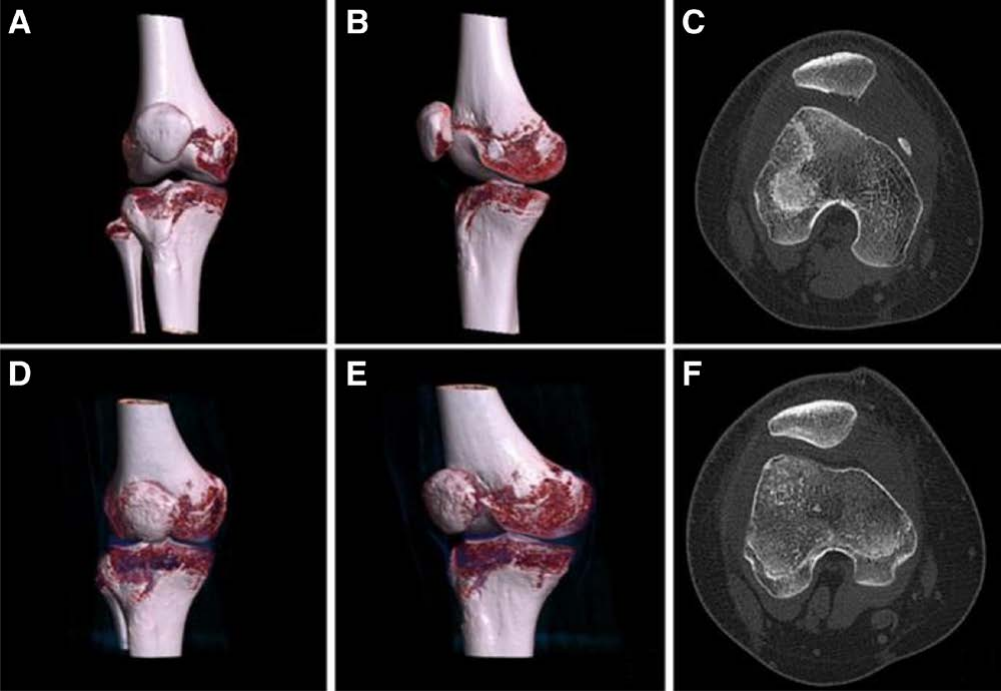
(A–C) Preoperative 3-dimensional CT reconstruction of the right knee. (D–F) Three-dimensional CT reconstruction of the right knee at 3 months after surgery.
